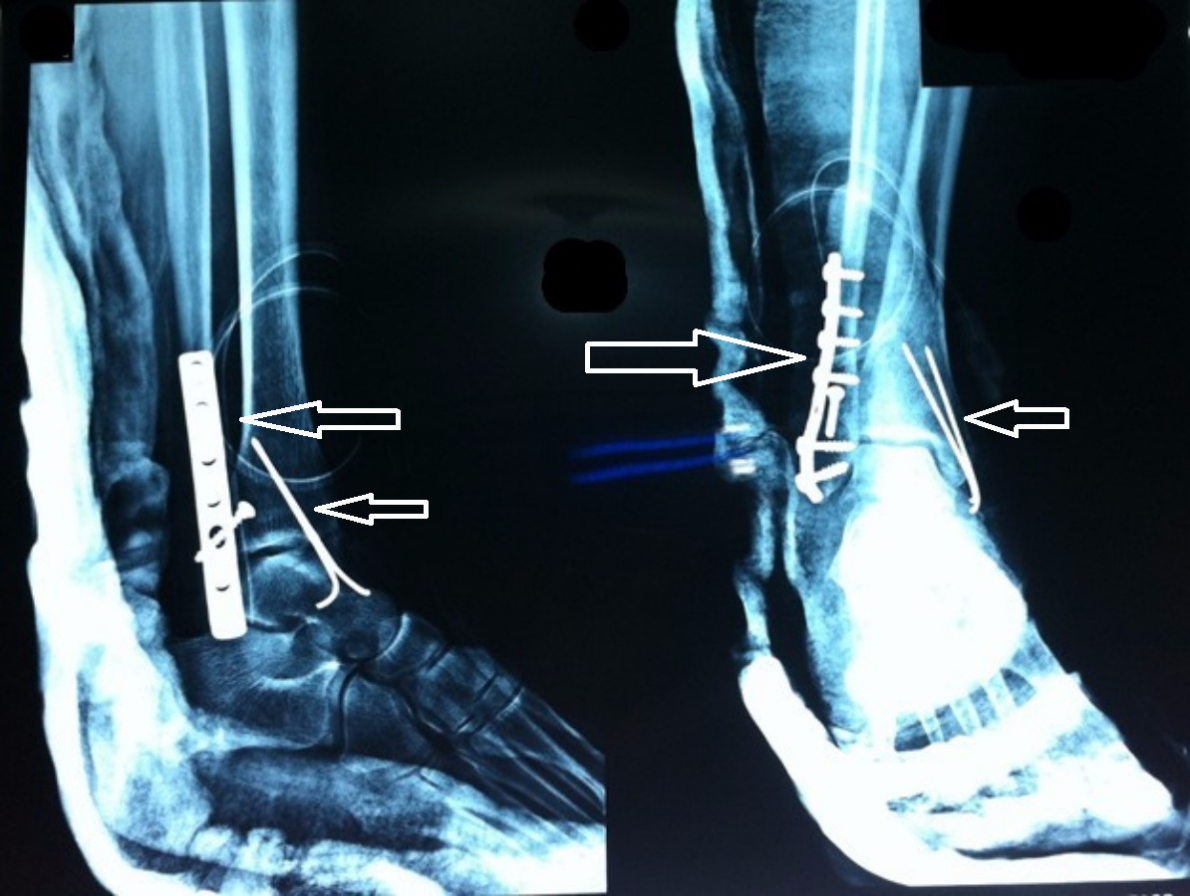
X-ray left foot After open reduction and internal fixation (white arrows).
